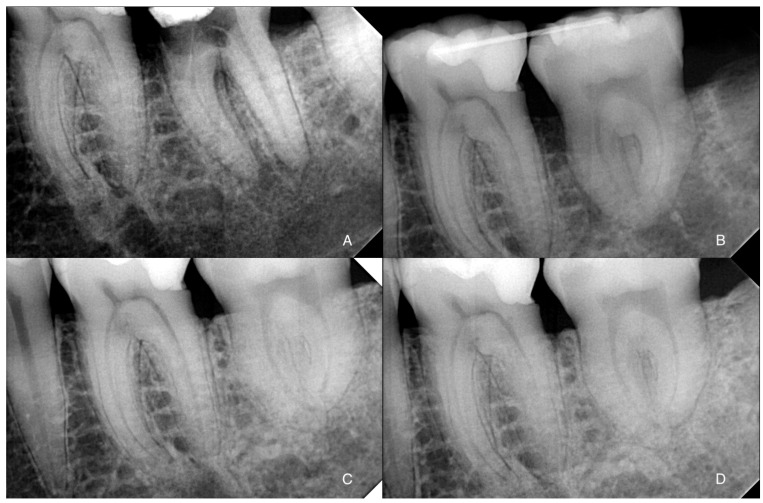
(A) X-ray showing the cervical root resorption of tooth 3.7; (B) X-ray showing the transplantation of 3.8 in 3.7 position immediately after the surgery; (C) X-ray showing the healing at 1-year follow-up; (D) X-ray at 3-year follow-up.
